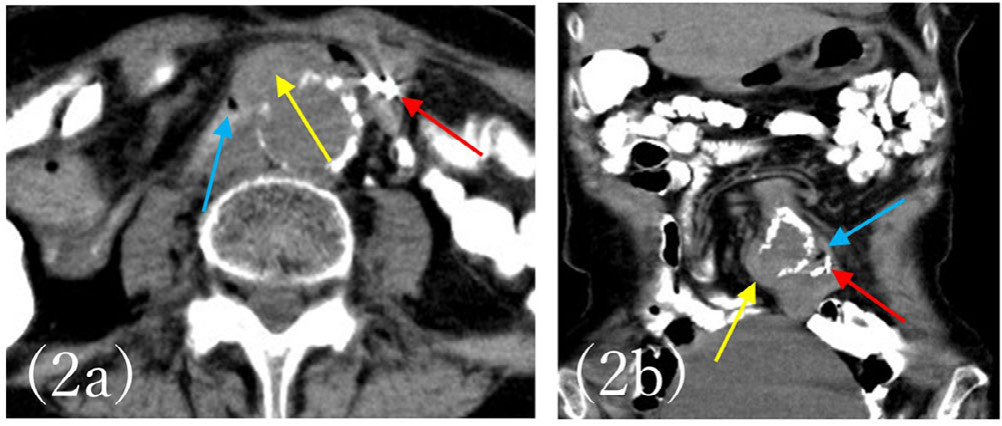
Axial plane (a) and coronal plane (b) of the computed tomography (scan showed the abdominal aortic aneurysm. A 35 mm aneurysm was found in the lower abdominal aorta at the bifurcation of the renal arteries. Blue arrows indicate free air, yellow arrows indicate saccular protrusion from the aorta toward the small intestine, and red arrows indicate marking clips.
